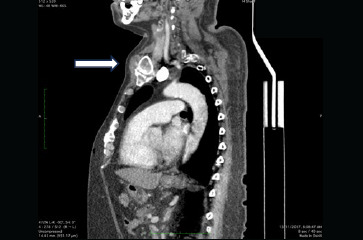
Sagittal view of the neck and thoracic CT scan showing soft tissue swelling at the sternoclavicular joint.
